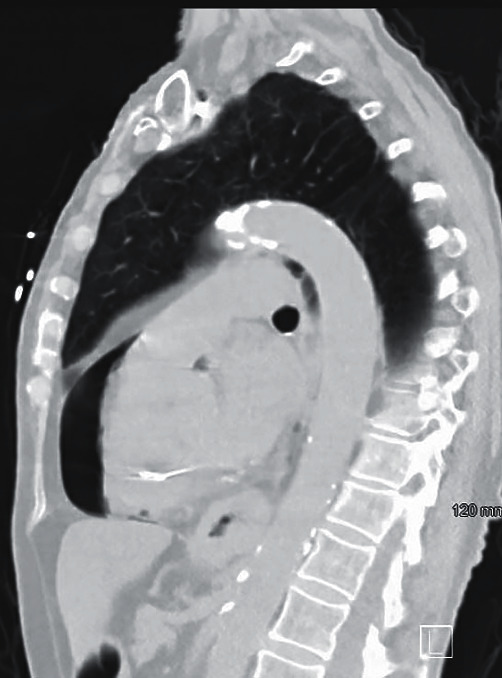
CT chest sagittal view demonstrating pneumopericardium.
